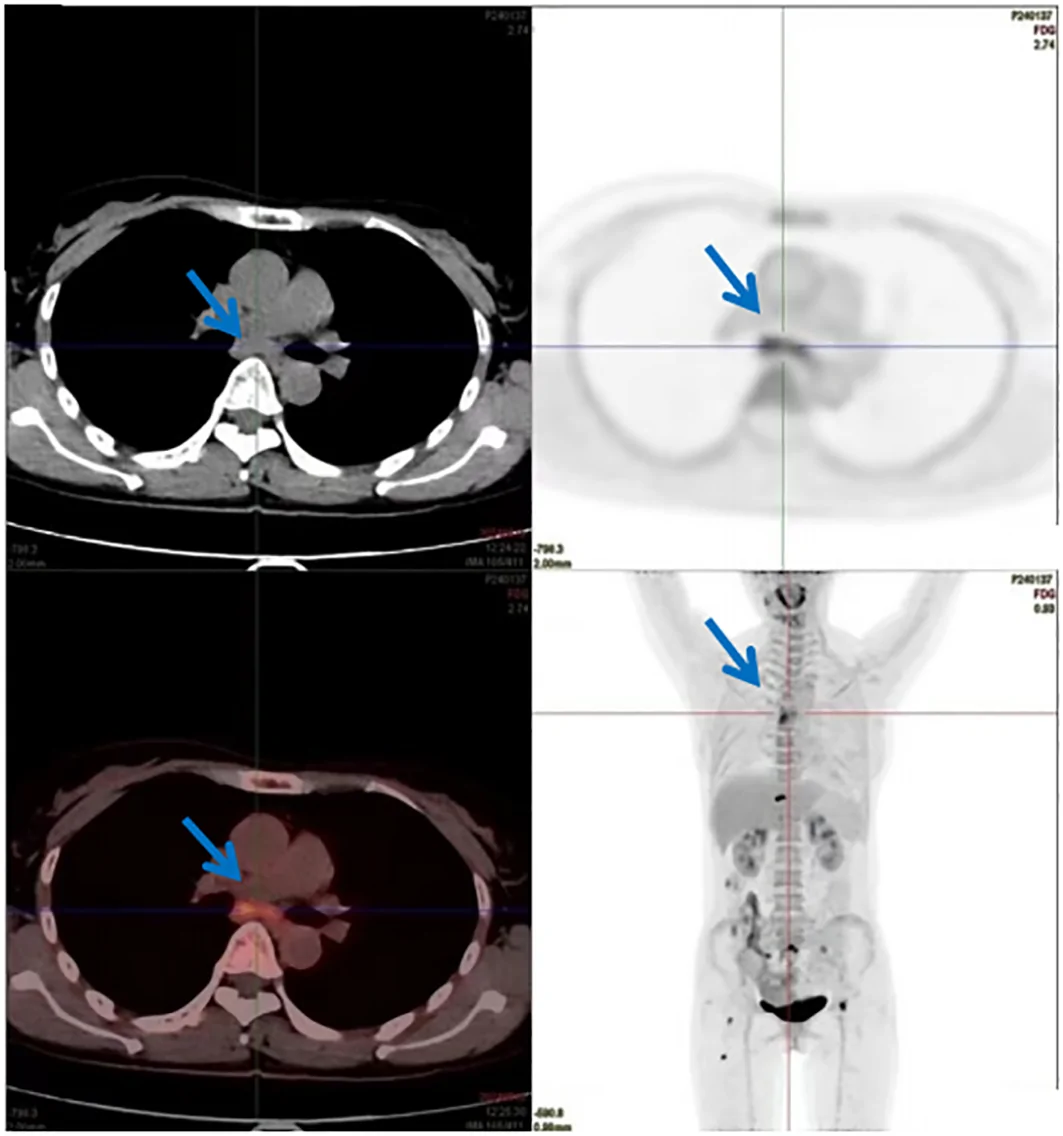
Axial CT, PET, fused PET/CT and whole-body PET maximum intensity projection (MIP) images. The blue arrow points to the enlarged mediastinal lymph node with high metabolic activity.
